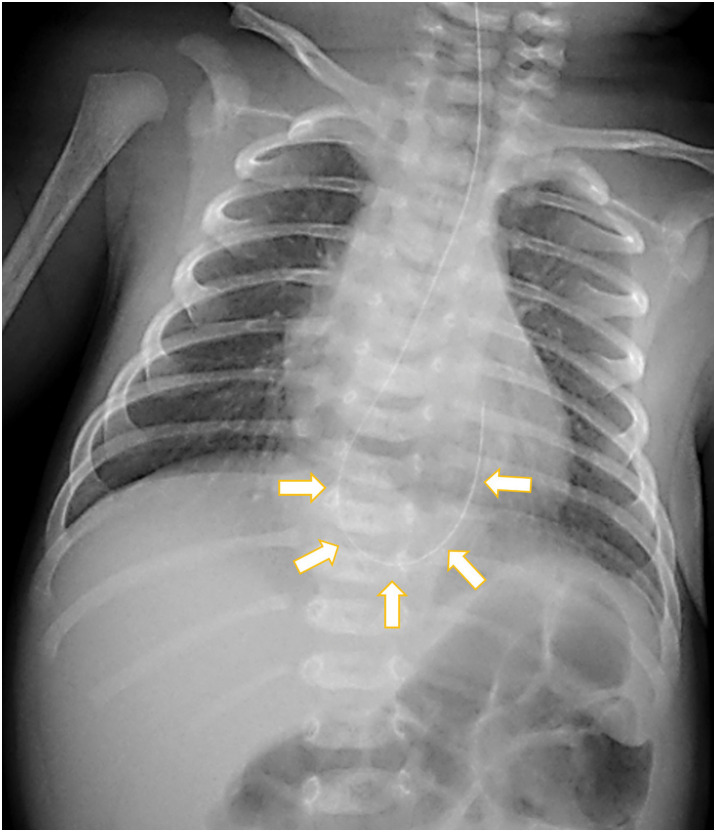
Chest radiograph. The orogastric tube curled at the level of diaphragm, which is mimicking so-called “coiled-up sign” (arrows).
